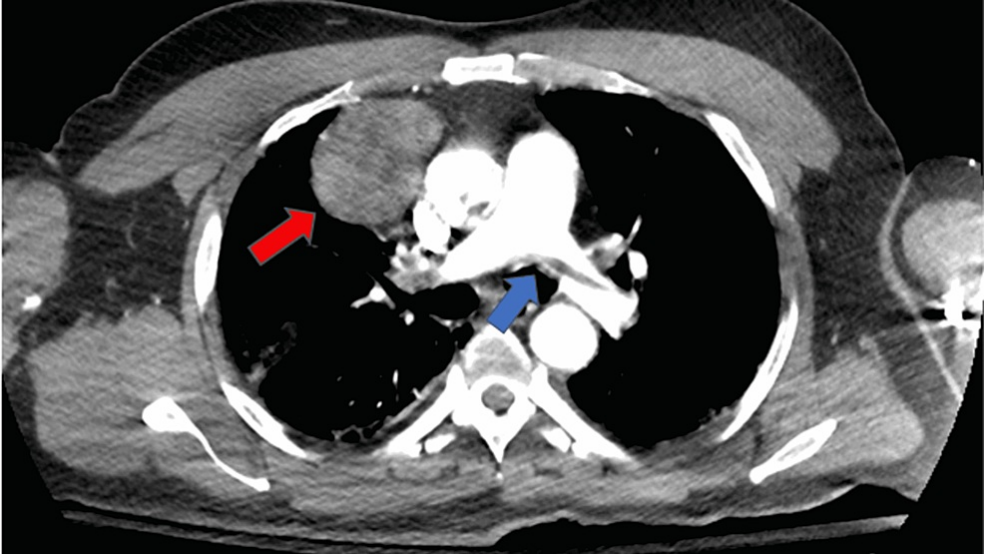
CT scan showing a right-sided anterior mediastinal mass measuring 6 cm x 4.8 cm x 7.1 cm (red arrow) and a thin saddle PE (blue arrow) PE: Pulmonary emboli.
